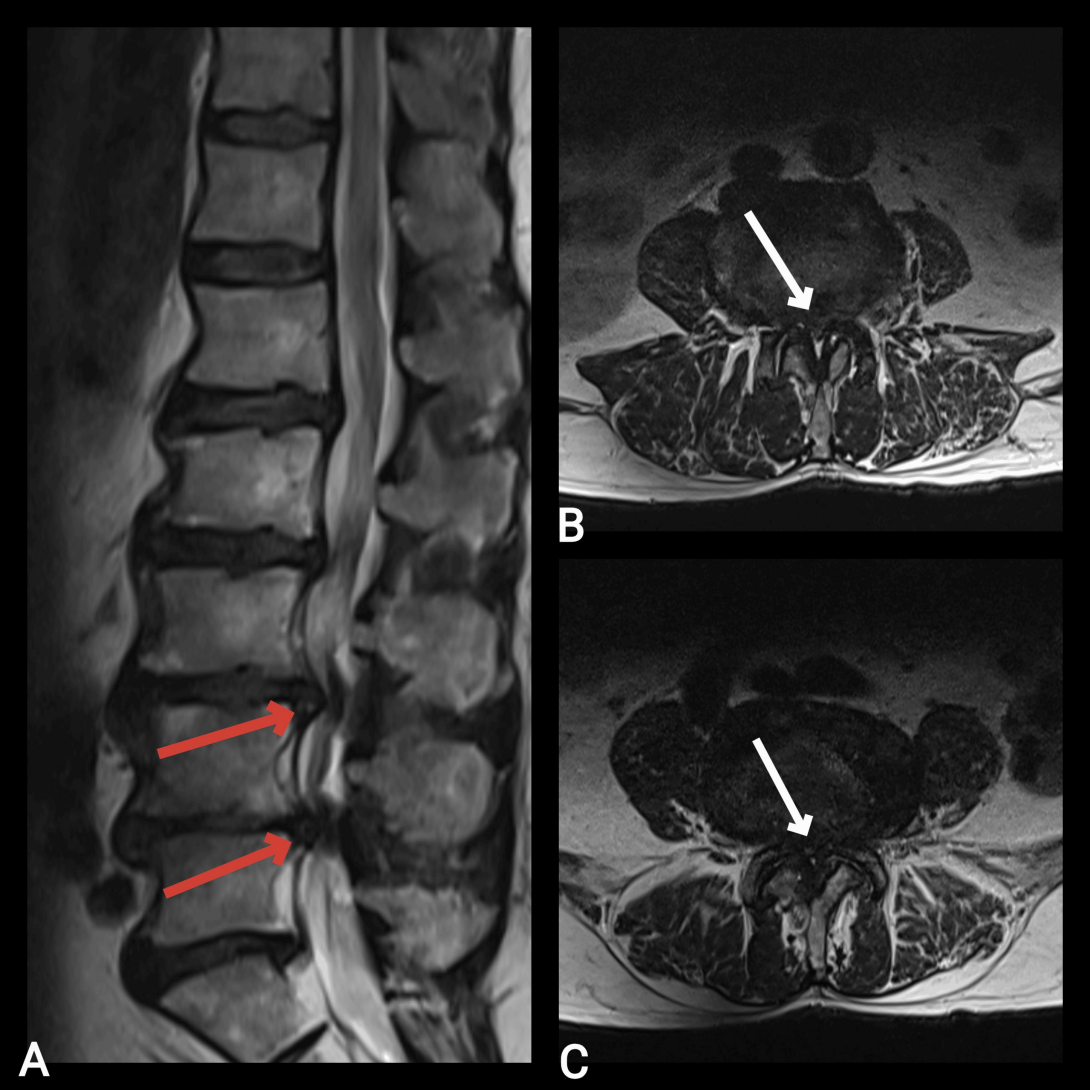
Preoperative MRI of an 81-year-old male showing high-grade spinal stenosis in the sagittal plane (red arrow) and axial plane (white arrow) at the L3-L4 and L4-L5 levels. A: T2 sagittal; B: T2 axial at the L3-L4 level; C: T2 axial at the L4-L5 level.
